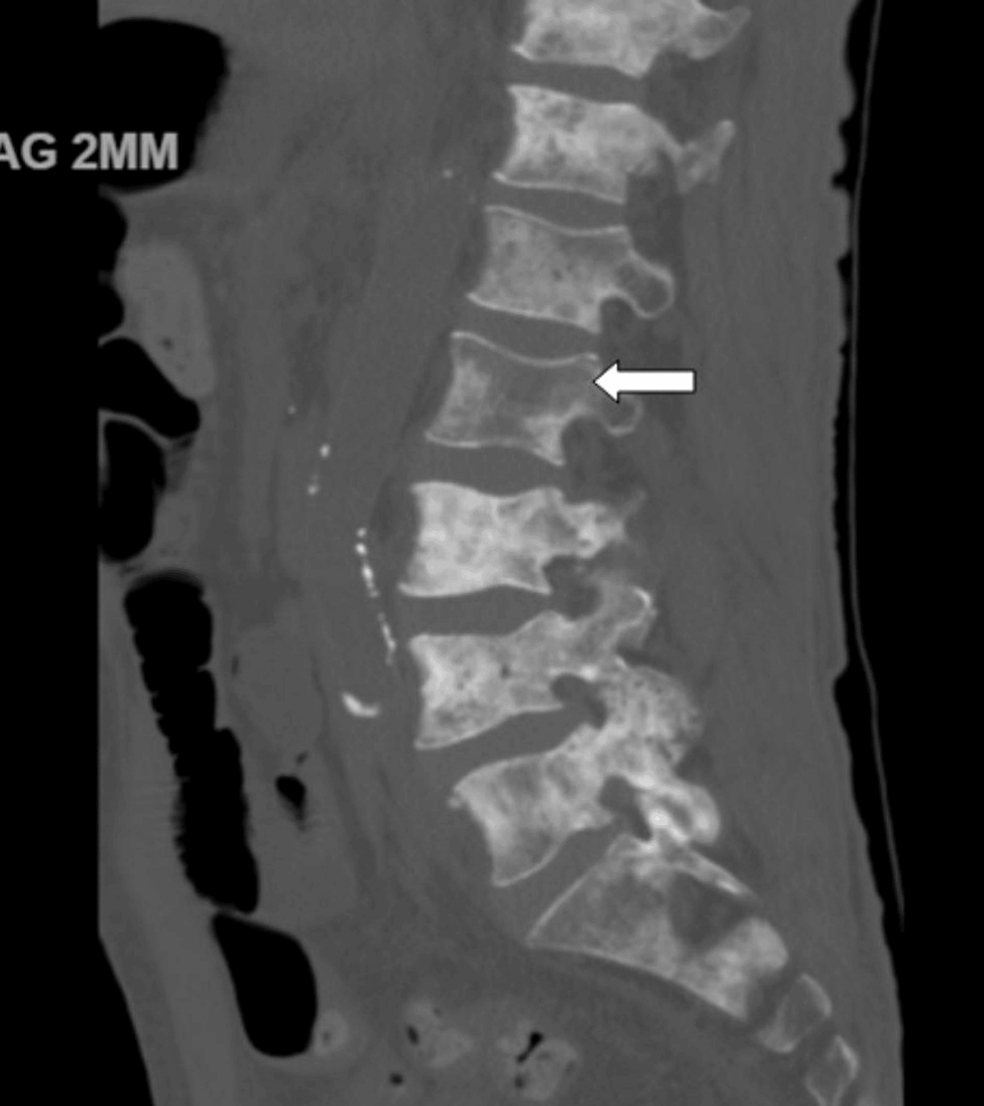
CT lumbar spine showing patchy sclerotic changed (white arrow)
